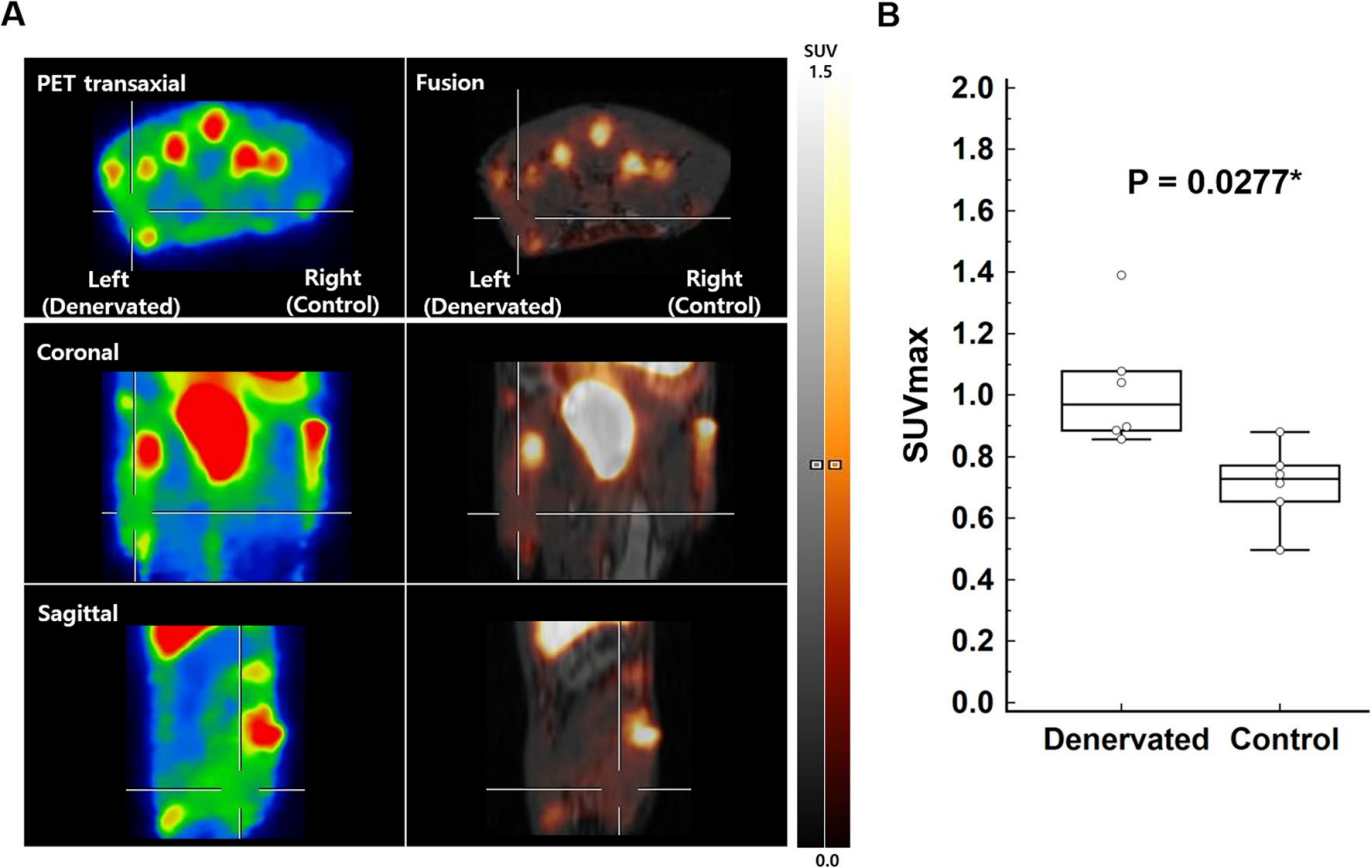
Denervation 3 week results of ^18^F-ASEM PET/MRI. A
